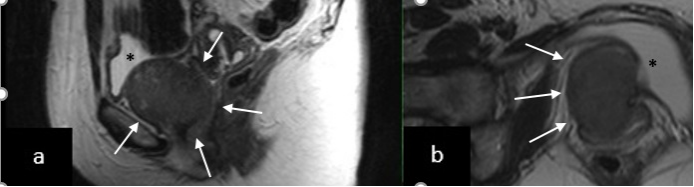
(# Case 3) MRI image of the 6cm vaginal leiomyoma occupying the upper portion of the anterior vaginal wall at (a) sagittal and (b) transverse planes (T2- weighted). The mass (multiple arrows) applies external pressure to the urinary bladder (indicated with an asterisk).
